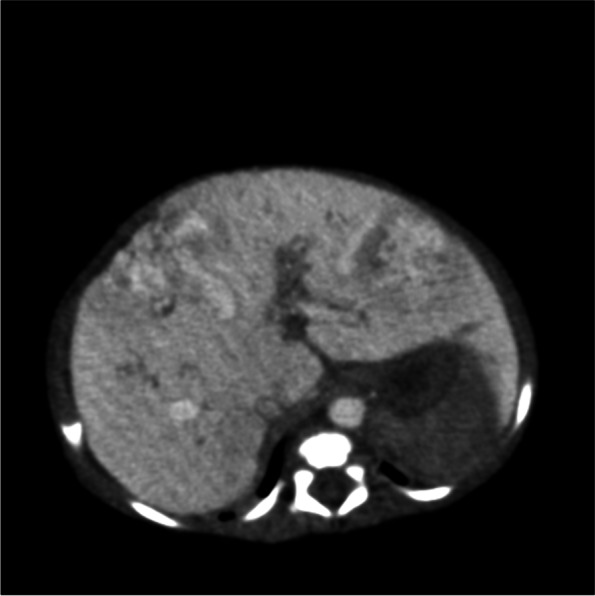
Computed tomography (CT) scans of a patient with Congenital hepatic hemangioma
